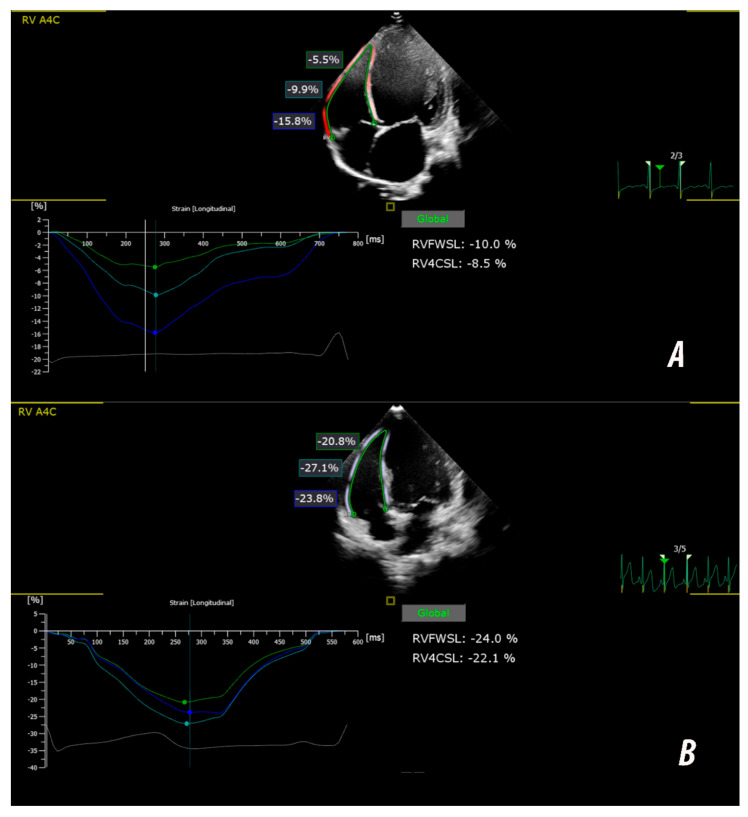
Representative examples of RV STE analysis in the apical four-chamber view. (A) Patient with DCM showing severely reduced right-ventricular deformation (RV free-wall longitudinal strain [RVFWSL] −10.0%, RV four-chamber strain [RV4CSL] −8.5%). (B) Age-matched healthy control with preserved right-ventricular deformation (RVFWSL −24.0%, RV4CSL −22.1%). Colored curves represent segmental strain profiles for basal, mid-ventricular, and apical free-wall segments, with the average global strain shown in white.
